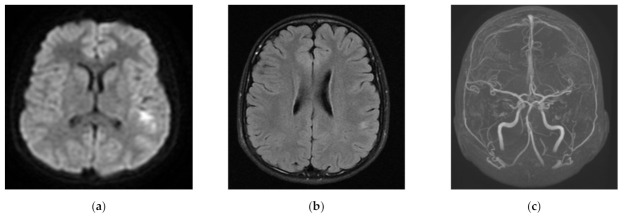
Discreet disseminated PACI (partial anterior circulation infarct) foci in left posterior temporal and parietal cortex/subcortical region: (a) MRI DWI (diffusion weighted imaging in magnetic resonance)—restricted diffusion in left posterior temporal/parietal cortex; (b) Flair MR—subtle signal changes in the same region; (c) MRA (magnetic resonance angiography)—occlusion of distal (cortical) branches of left MCA.
